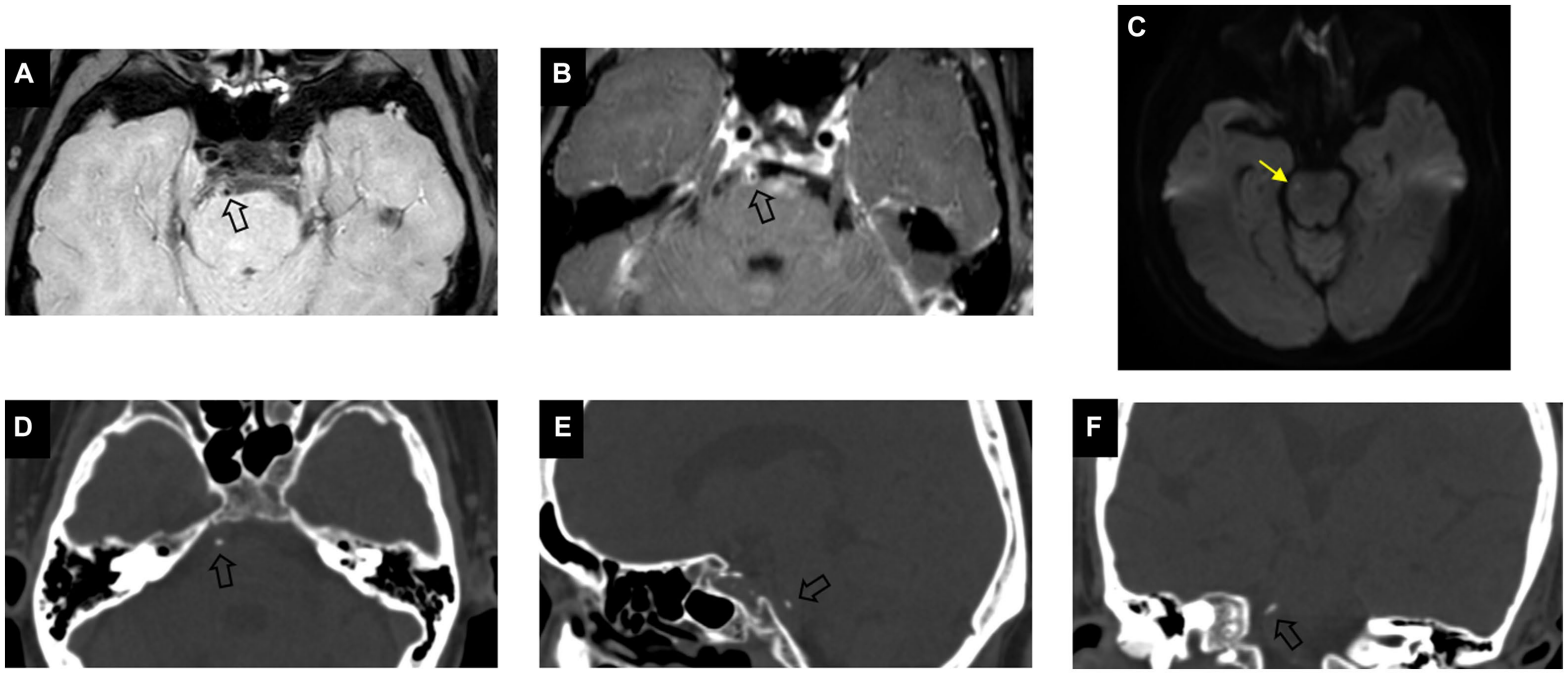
Example of calcification presence in a culprit plaque of a 64-year-old male symptomatic patient. Pre-contrast (A) and post-contrast (B) vessel wall MRI show an eccentric plaque (arrow) in the basilar artery. DWI image (C) demonstrates the presence of hyperintensity (yellow arrow) in the brainstem. Axial (D), sagittal (E), and coronal (F) CT images demonstrate the corresponding presence of calcification (arrow) which is classified as single, spotty, and intimal predominant calcification on visual assessment.
